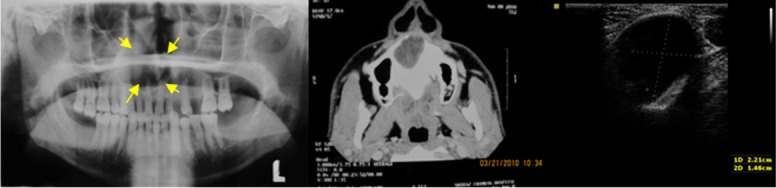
A case of radicular cyst. (A) Panoramic view reveals a radiolucent lesion on the anterior right side of the maxilla. (B) CT scan (tissue level) shows lytic lesion in the maxilla. (C) Ultrasound image shows anechoic lesion without internal echoes.
